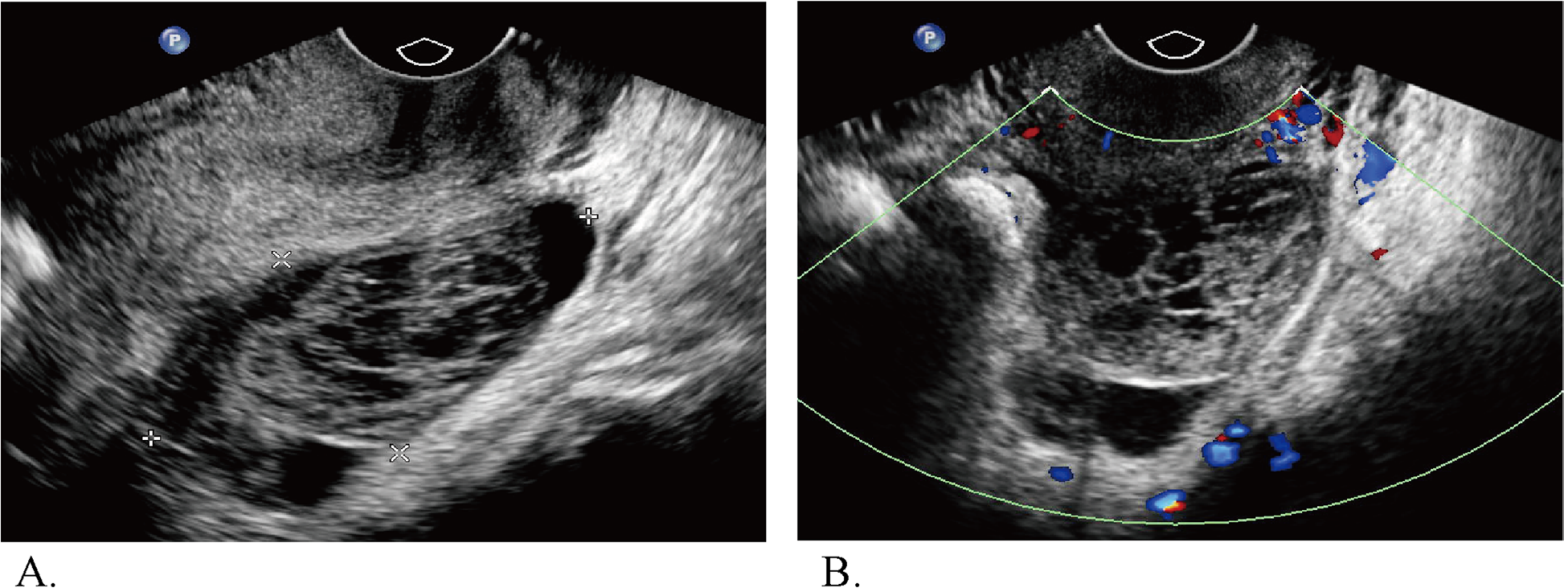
Ultrasound images of a 31-year-old woman with a pathologically proven hemorrhagic cyst and a CA 125 level of 28 U/ml. A Transvaginal ultrasound revealed a 6.5-cm multilocular cyst with no solid component in the left adnexa. B Color Doppler ultrasound revealed no color flow (color score = 1). The lesion was categorized as IOTA SR B4 and B5 and O-RADS 3 based on the sonographic findings
